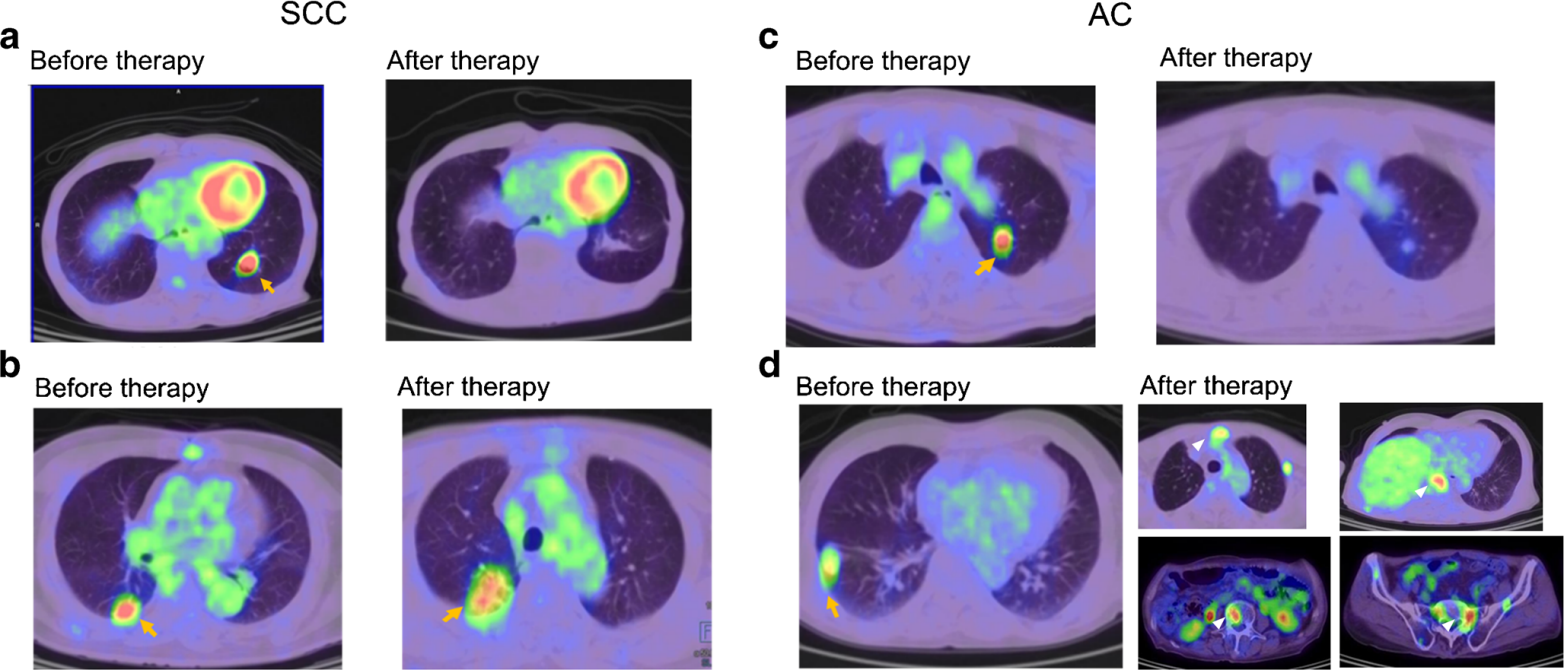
Axial FDG PET/CT fusion images of representative cases of stage I SCC and stage I AC with lung tumor lesions indicated by yellow arrows, and bone metastases indicated by white arrowheads. SCC patients: a 80-year-old male having tumor with SUVmax 10.34 and MTV 5.78 cm^3^, with 48.6 months PFS/OS, and b 76-year-old male having tumor with SUVmax 7.04 and MTV 7.96 cm^3^ with 5.5 months PFS and 27.1 months OS; AC patients: c 77-year-old male having tumor with SUVmax 5.85 and MTV 1.59 cm^3^ with 55.4 months PFS/OS, and d 73-year-old male having tumor with SUVmax 4.28 and MTV 2.51 cm^3^ with 17.2 months PFS and 29.7 months OS. Multiple bone metastases are observed in case “d,” shown by white arrowheads in after-therapy images; upper row—left: sternal, right: thoracic vertebra; lower row—left: lumbar vertebra, right: sacrum. In both SCC and AC, MTV correlated better than SUVmax with survival outcomes
